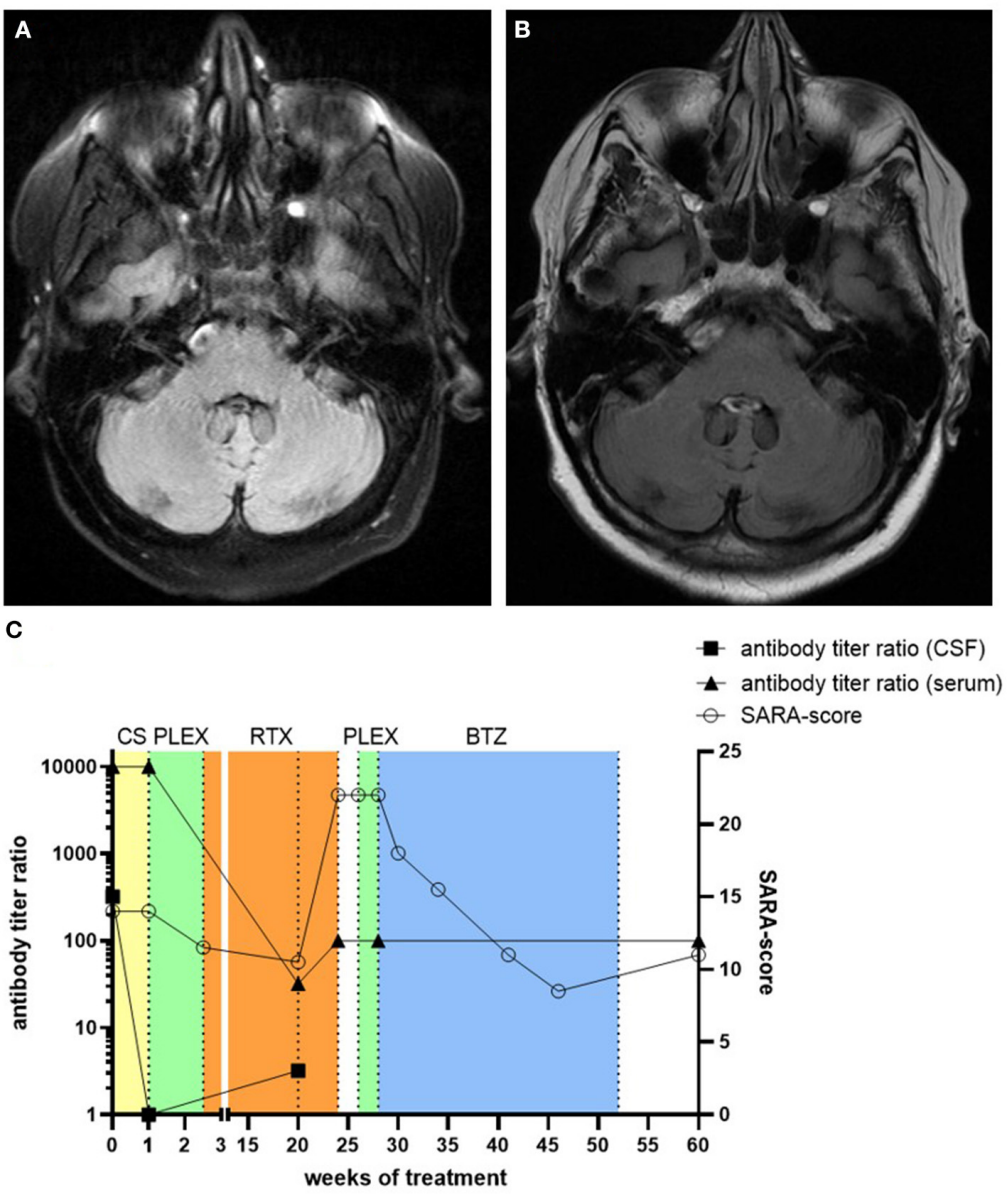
Native axial FLAIR MR-images of the patient 4 months after symptom onset (A) and after clinical deterioration 5 months after treatment with rituximab (B). No cerebellar atrophy or inflammatory lesions were detected. The clinical course of the patient at different time-points with corresponding treatment regimens, SARA-score values, and antibody titer ratio in both CSF and serum is depicted (C). CS, corticosteroids; RTX, rituximab; PLEX, plasma exchange; BTZ, bortezomib.
